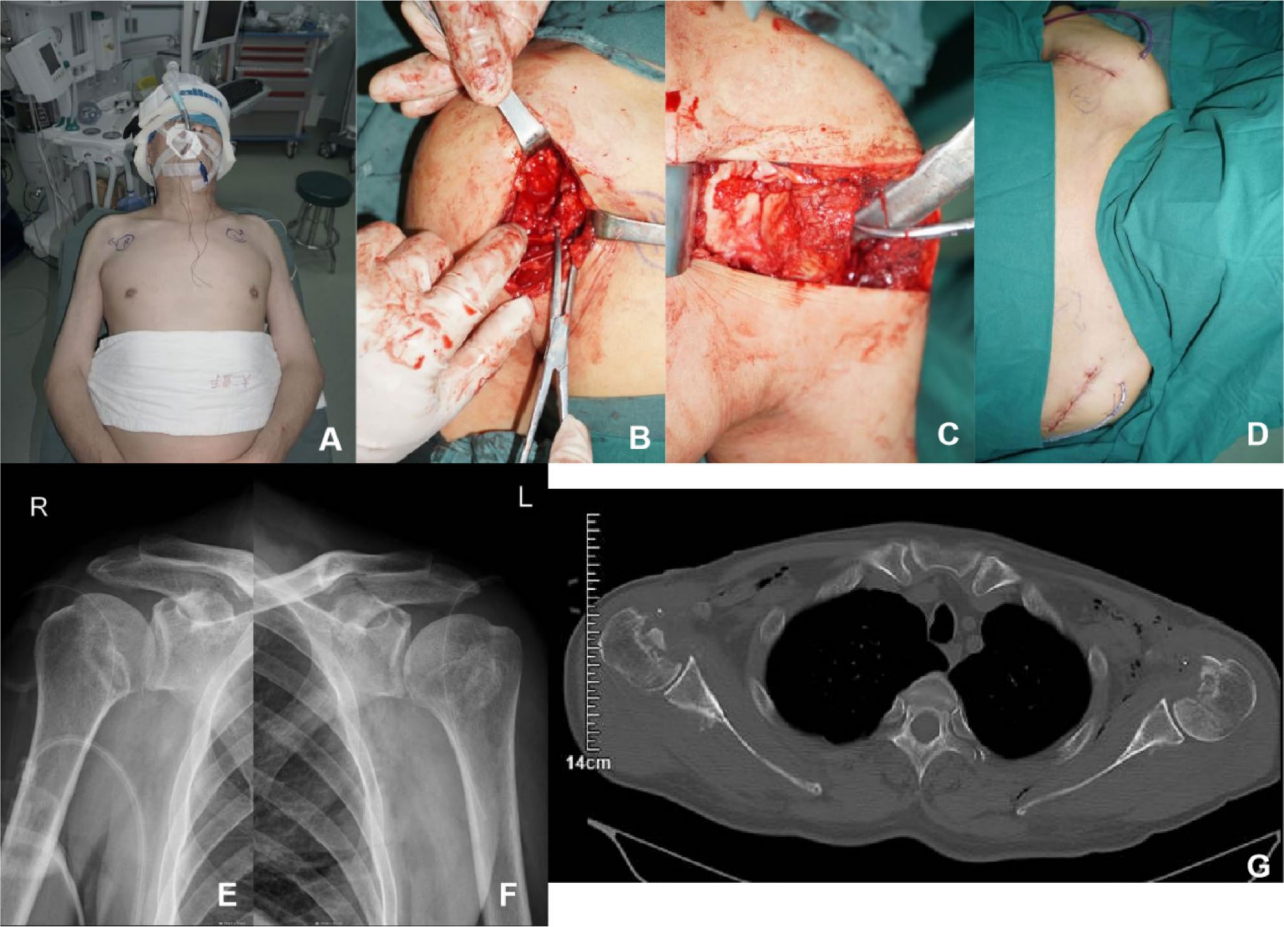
Intraoperative and postoperative images of Case 1. A Beach-chair position; B Articular surface defect of the right humeral head; C Articular surface defect of the left humeral head; D Incisions of both shoulders; E Postoperative AP view of the right shoulder; F Postoperative AP view of the left shoulder; G Postoperative CT scans of both shoulders
